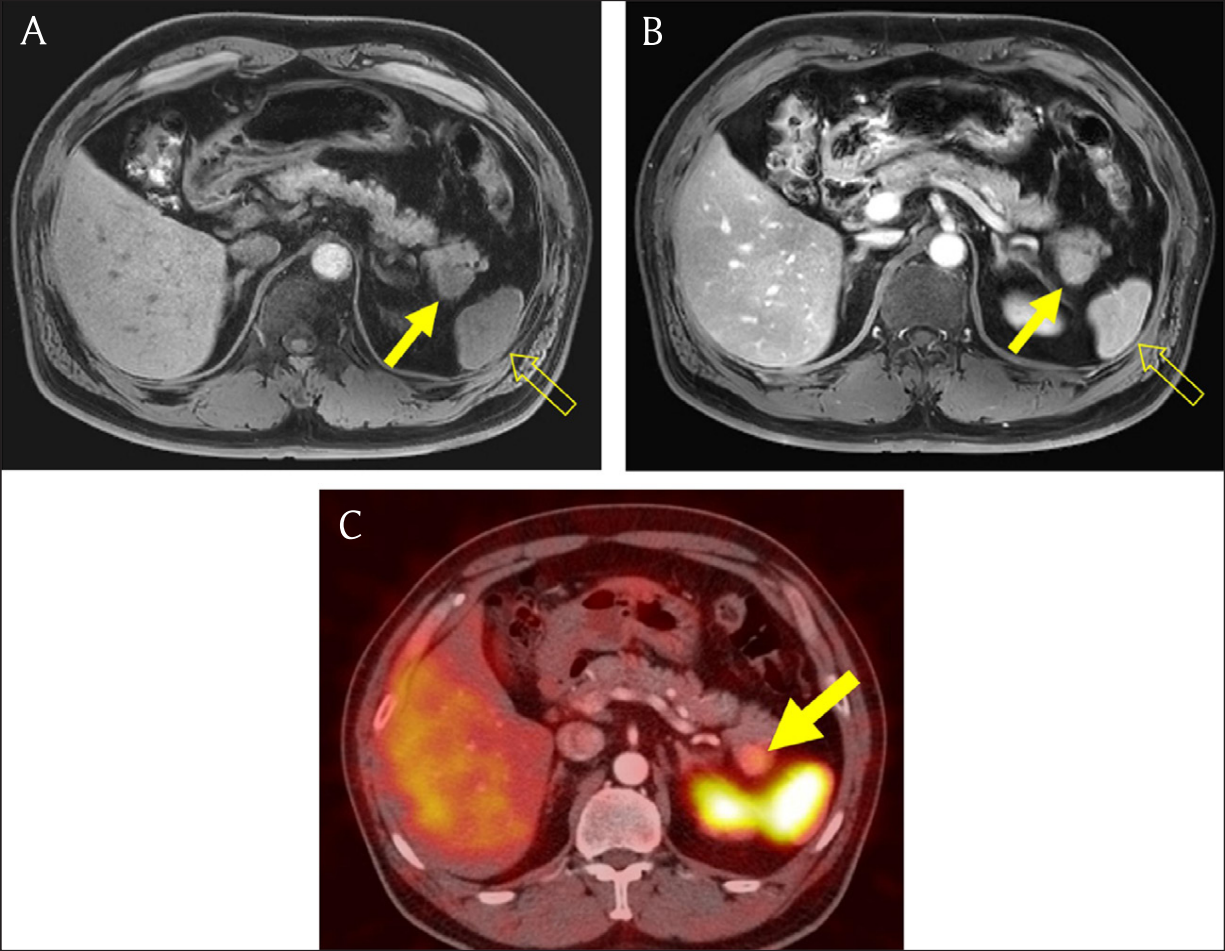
A 52-year-old man with accessory spleen in pancreatic tail. (A) Axial fat-suppressed T1-weighted image shows a mass-like lesion (arrow) with similar signal intensity compared with the spleen (open arrow) in pancreatic tail. (B) On axial gadolinium-enhanced portal venous phase MR image, the enhancement of the pancreatic mass (arrow) was similar to that of the spleen (open arrow). (C) Heat-damaged red blood cell scintigraphy shows intense uptake in the mass (arrow).
